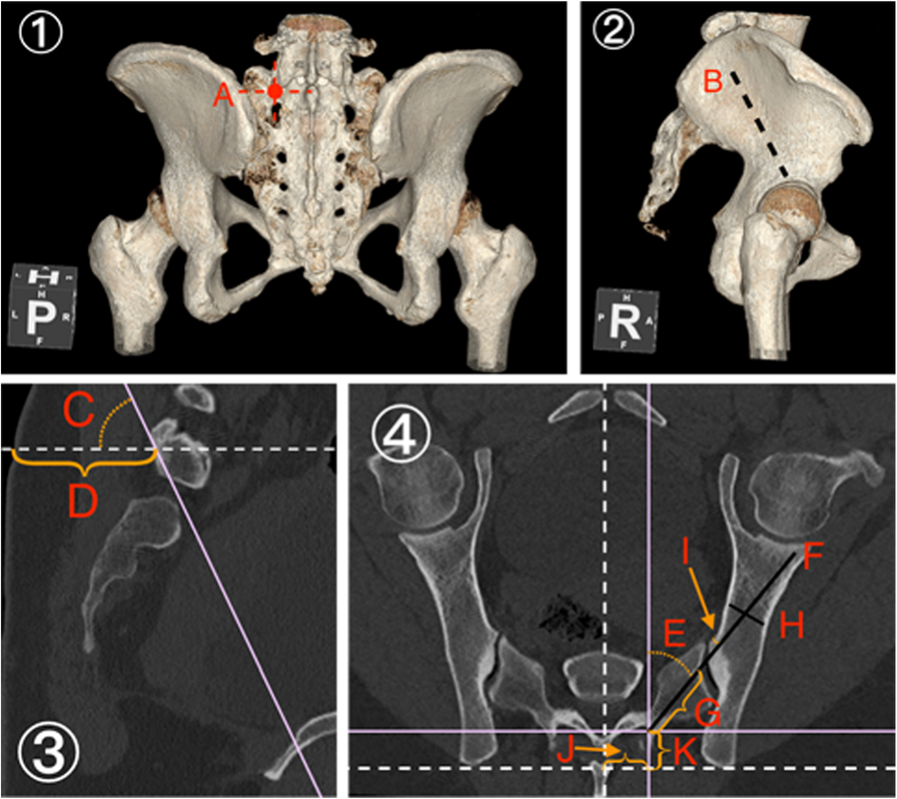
Case 2, 55-year-old female. 1 Posterior view of CT 3D imagine. A The entry point of S1AI trajectory. 2 Lateral view of CT 3D imagine. B The direction of S1AI trajectory. 4 Sagittal reconstructed plane of S1AI trajectory. C Sag. A: 62.0°; D Skin D.: 59.1 mm. 4 Transverse plane of S1AI trajectory. E Tsv. A.: 37.4°; F Max. L.: 121.1 mm; G Sac. L.: 43.1 mm. H Iliac W.: 14.5 mm; I Cortex D.: 2.0 mm; J Mid. D.: 24.7 mm; K PSIS D.: 19.8 mm
